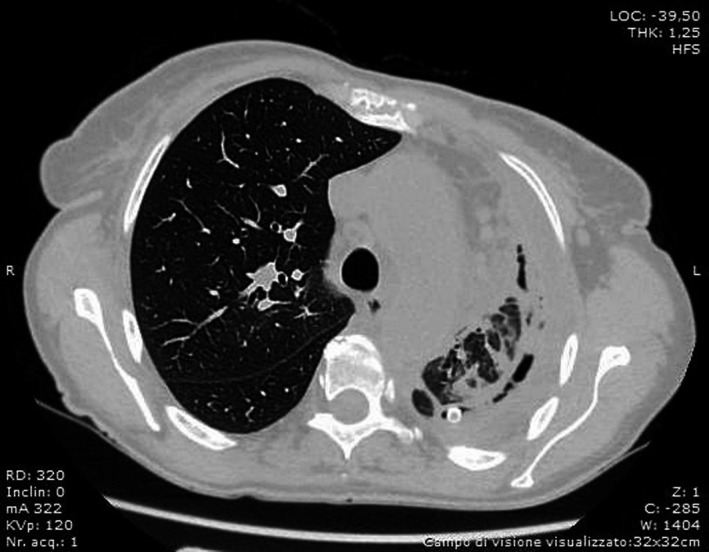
CT scan shows decreasement of pleural effusion with atelectasis of lower left lobe and diffuse pleural thickening
